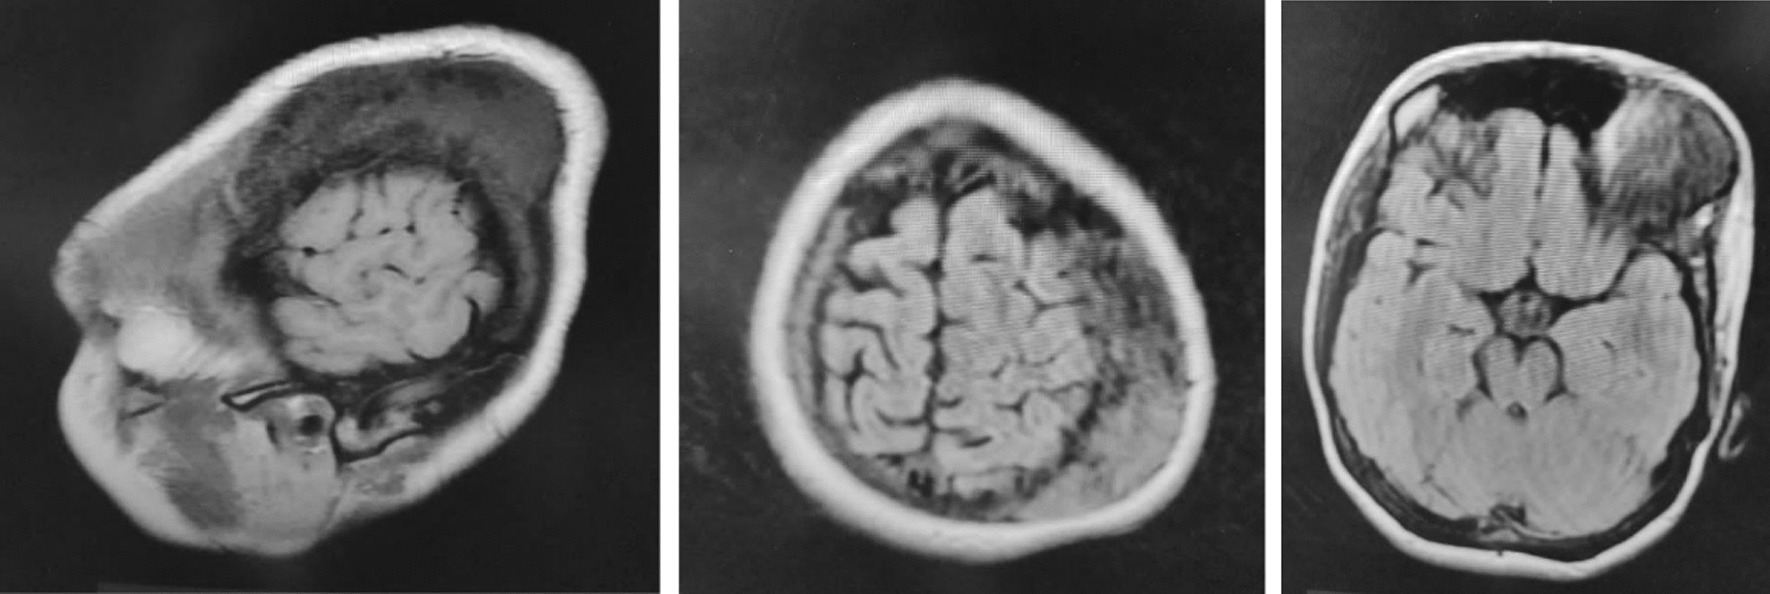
MRI showing the heterogeneous bony tumor in the left frontoparietal region; the brain was within normal parameters
